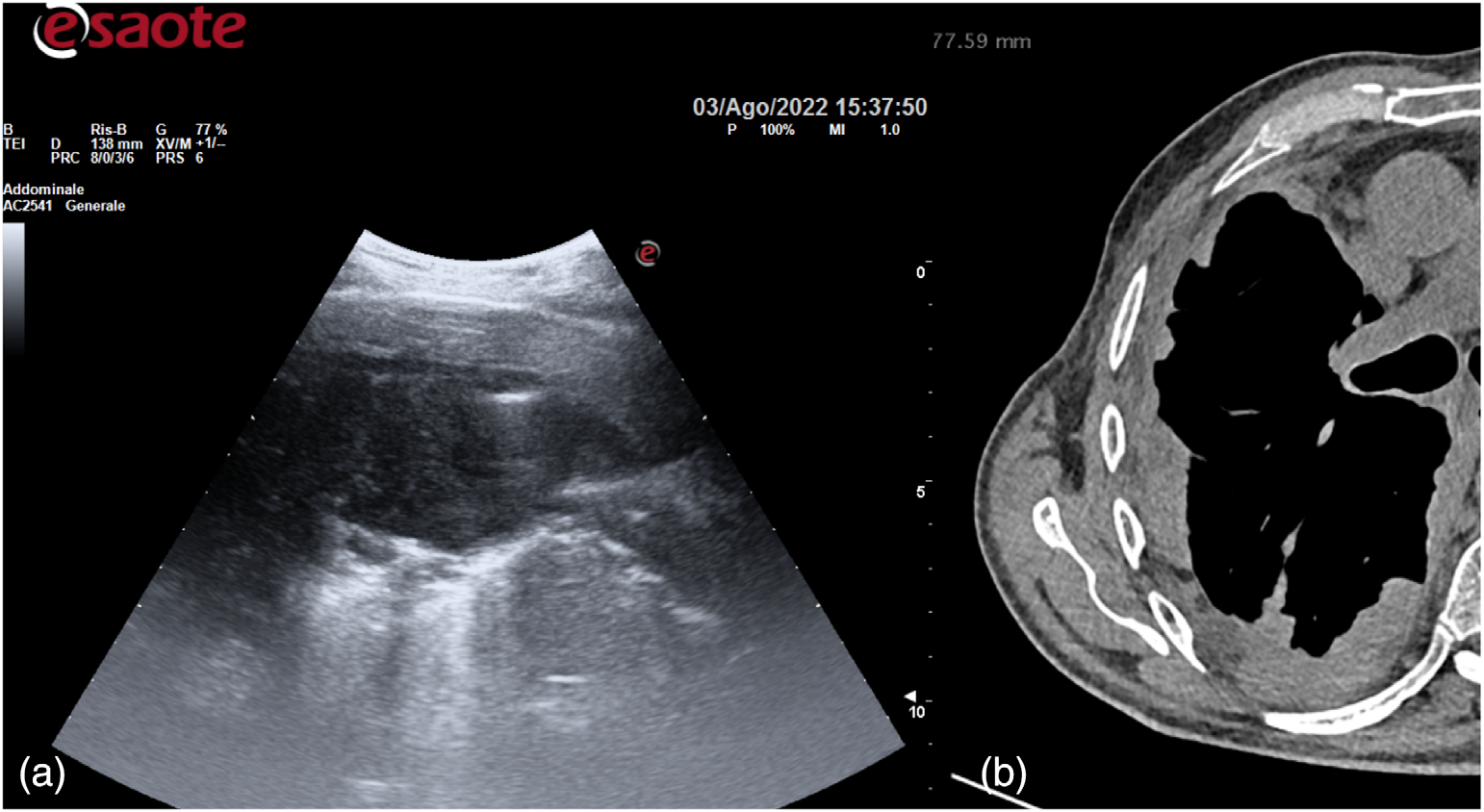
(a) An ultrasound study can easily define the precise pleural thickening localization, local infiltration, mass extent, its nature (solid, cystic or complex) and ultrasound features. (b) Thoracic computed tomography (CT) pleural circumferential thickening.
